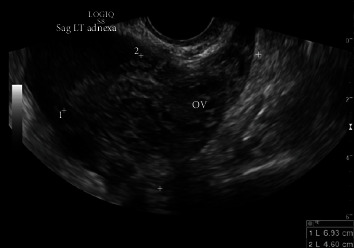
Transvaginal ultrasound image of left adnexa. OV depicts ovarian tissue. Complex left adnexal mass measuring 6.9 × 4.6 × 4.7 cm^3^.
